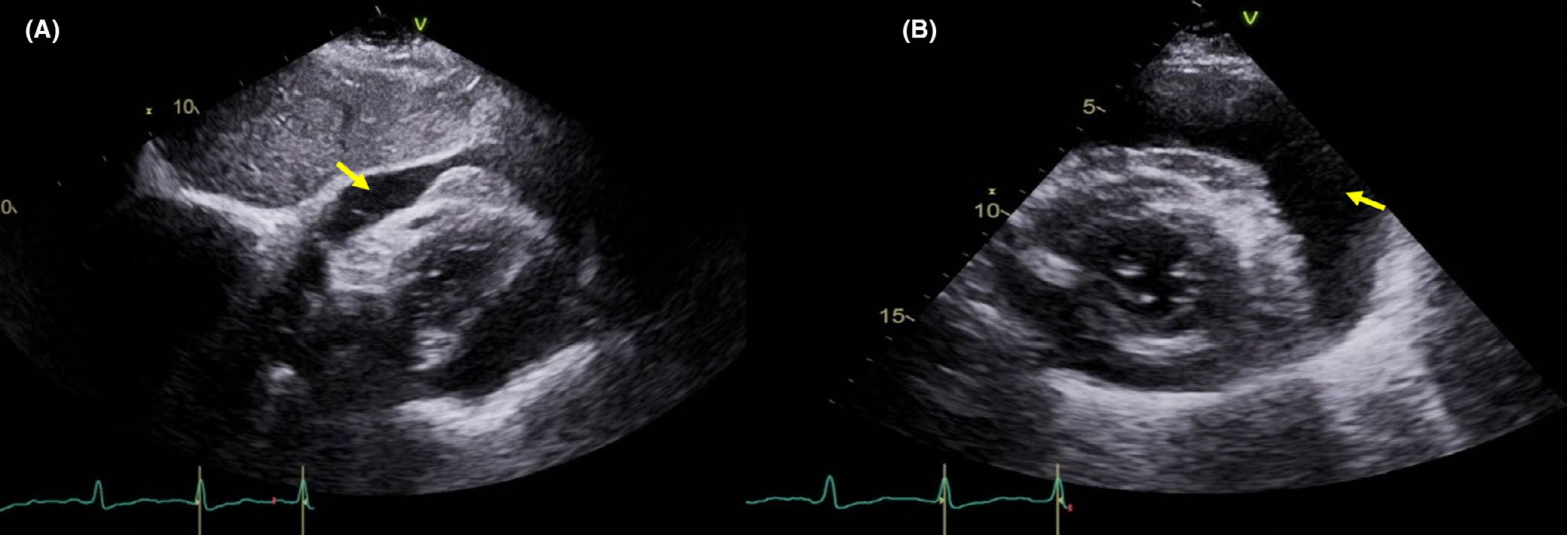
Transthoracic Echocardiography (TTE) on admission indicates large pericardial effusion (yellow arrow) seen on a subcostal view (A) and Short axis view (B)
